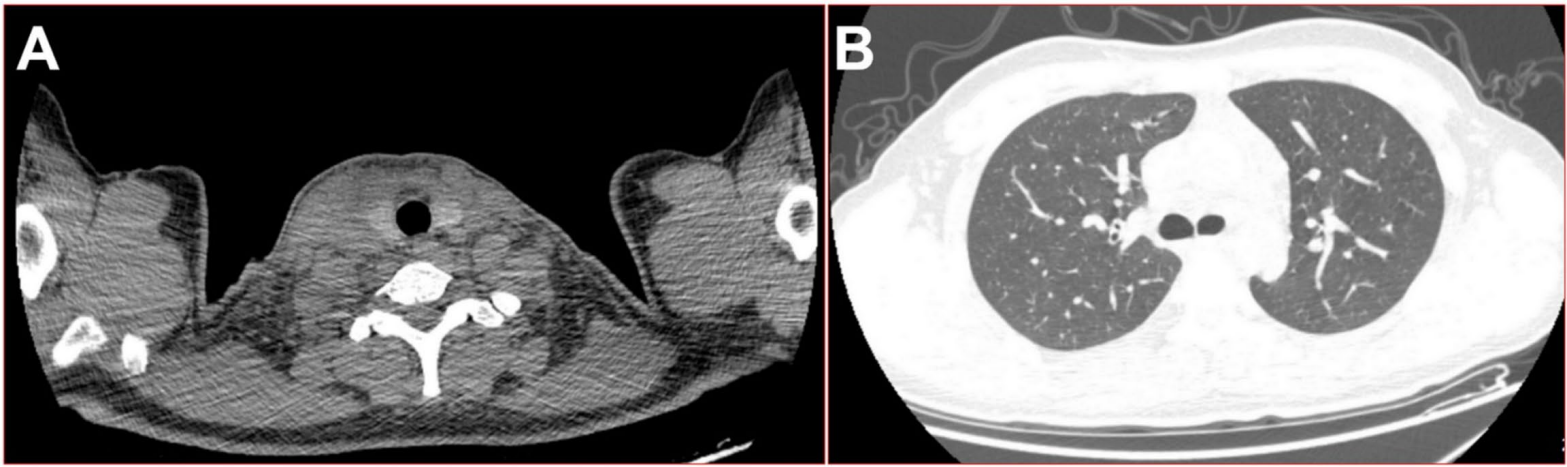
On the fourth day of the fever, 16-slice computed tomography (CT) images of the patient were obtained. (A) Swelling of the right side of the neck soft tissue; (B) lung window in the upper thoracic cavity.
